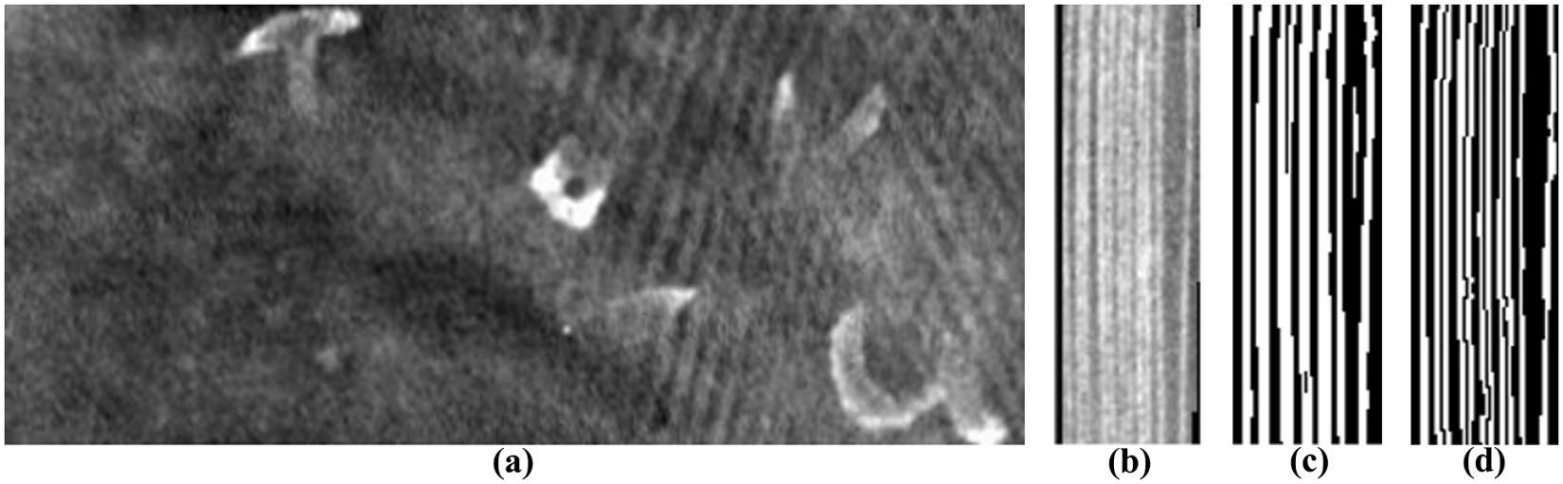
(a) Exemplary X-ray volume *xy*-slice of the scanned book: The high resolution and the orientation of the pages in the volume make it impossible to read each page separately with the naked eye. Multiple pages are present in one slice such that a virtual flattening is necessary. (b) *yz*-slice of the volume–the pages are separated by air gaps and look like small vessels. (c) The same *yz*-slice after applying the volume binarization step–the pages are binarized but still contains overlaps and gaps. (d) The final output after page segmentation for the *yz*-slice of (b)–overlapping pages are separated and gaps are closed.
